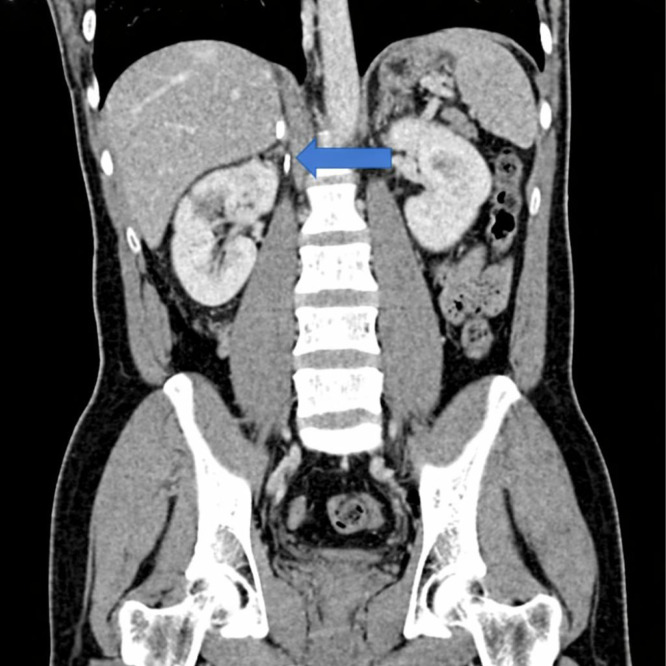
Contract enhanced CT showing surgical clips in the right adrenalectomy bed, with no soft tissue lesions around it.
